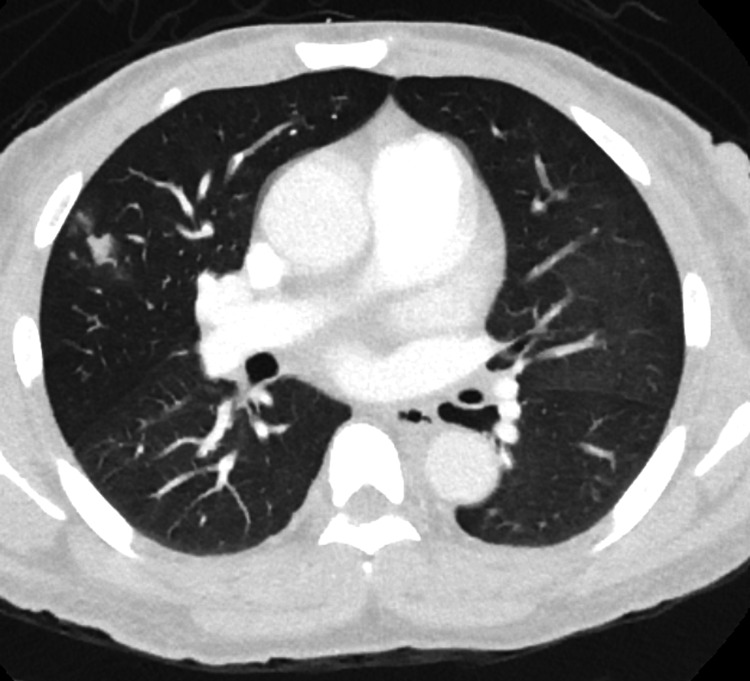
Computed tomography showing multifocal ground-glass and patchy consolidative opacities Computed tomography pulmonary angiography demonstrating multifocal ground-glass and patchy consolidative opacities without evidence of pulmonary embolism
